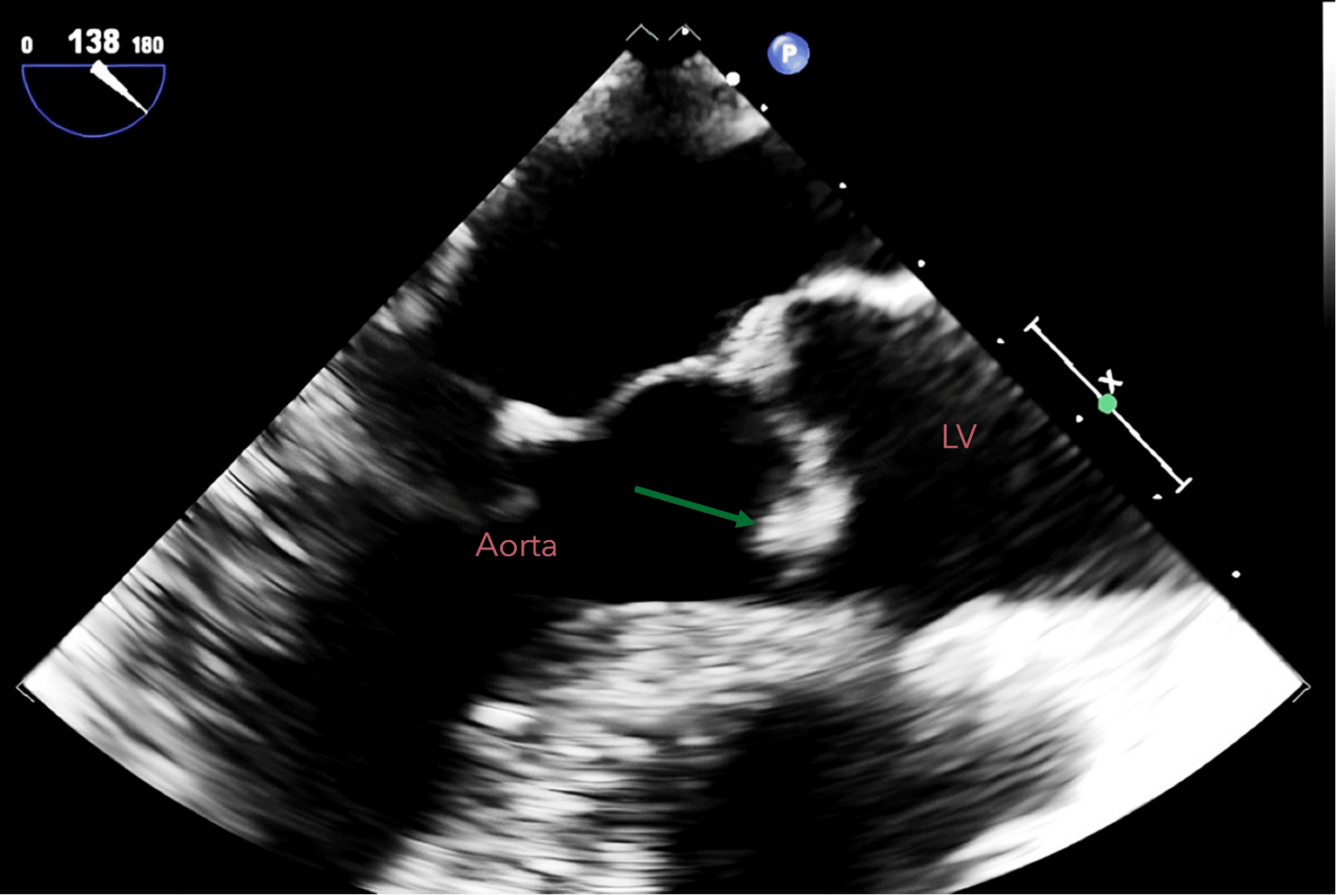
Echocardiography showing a 2-mm vegetation on the right coronary cusp of the aortic valve (green arrow)
